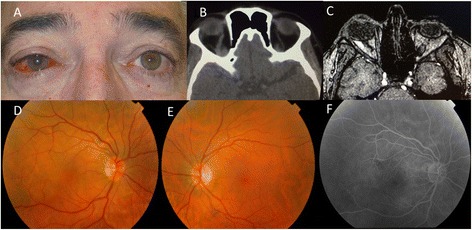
a Initial photograph showing tortuous and dilated episcleral vessels of the patient’s right eye. b CT shows an enlarged right superior ophthalmic vein. c MRA suggested a reduction of blood flow, which led to the suspicion of cavernous sinus-dural arteriovenous fistula. d Fundus photography of the right eye with venous congestion of the retinal veins and macular subretinal fluid. e The normal left eye. f Fluorescein angiography without clear leakage in the area of the serous detachment
